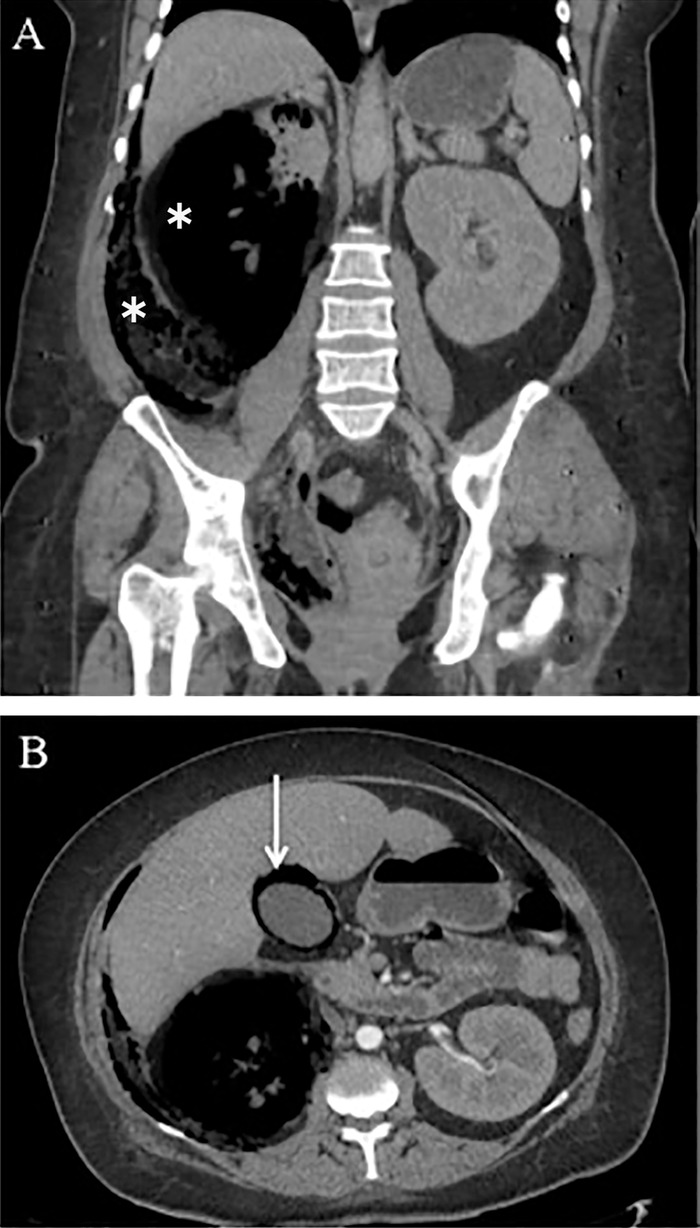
(A) Coronal view of contrast-enhanced computed tomography (CT) scan shows extensive parenchymal destruction of the right kidney (top asterisk) and gas extending through the retroperitoneum (bottom asterisk). (B) Axial view of contrast-enhanced CT scan shows intramural gas in the gallbladder (arrow).
